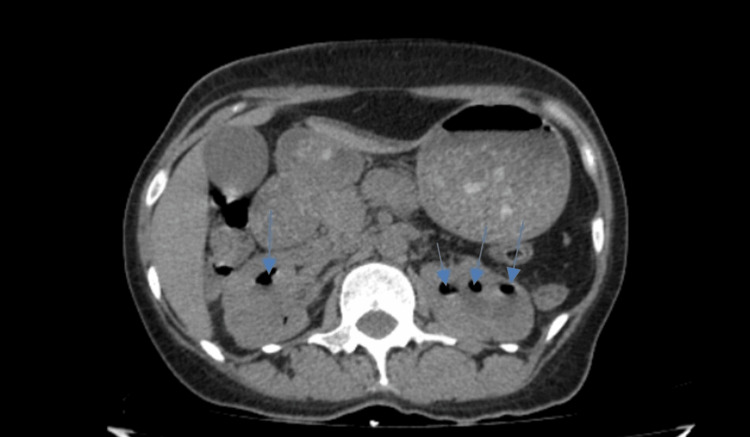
The CT scan shows bilateral emphysematous pyelonephritis before antibiotic treatment
